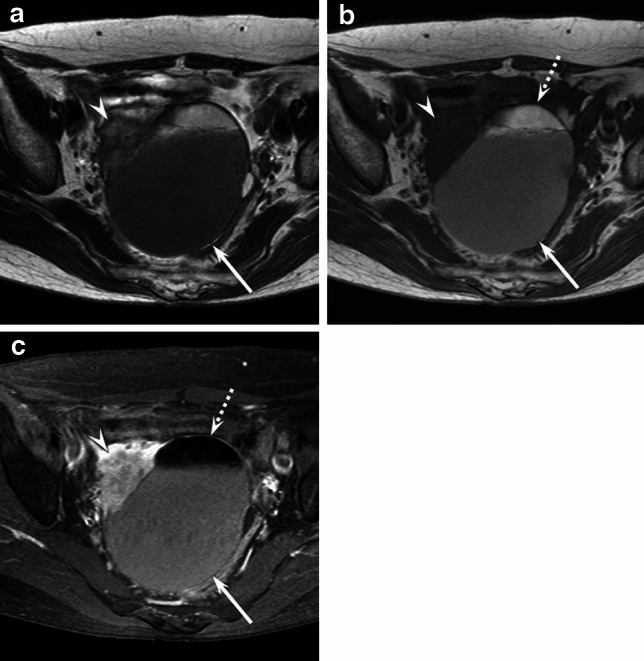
A 70-year-old woman with MT-MCT (stage IC1). a. Axial T2-weighted image showing a fat-containing mass (arrow) with exophytic sessile solid components and irregular margin (arrowhead). b. Axial T1-weighted image showing a fat-containing mass (arrow) with exophytic sessile solid components (arrowhead). The occupancy rate of fat components accounting for 6% (dotted arrow). c. Axial fat-suppressed contrast-enhanced T1-weighted image showing a fat-containing mass (arrow) with fat-suppressed area (dotted arrow) and exophytic sessile solid components (arrowhead)
